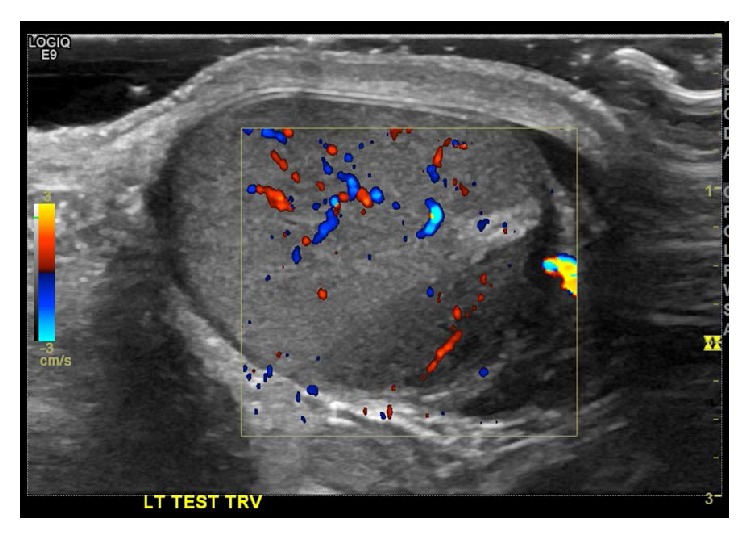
Testicular ultrasound showing hypoechoic lesion in left testis.
